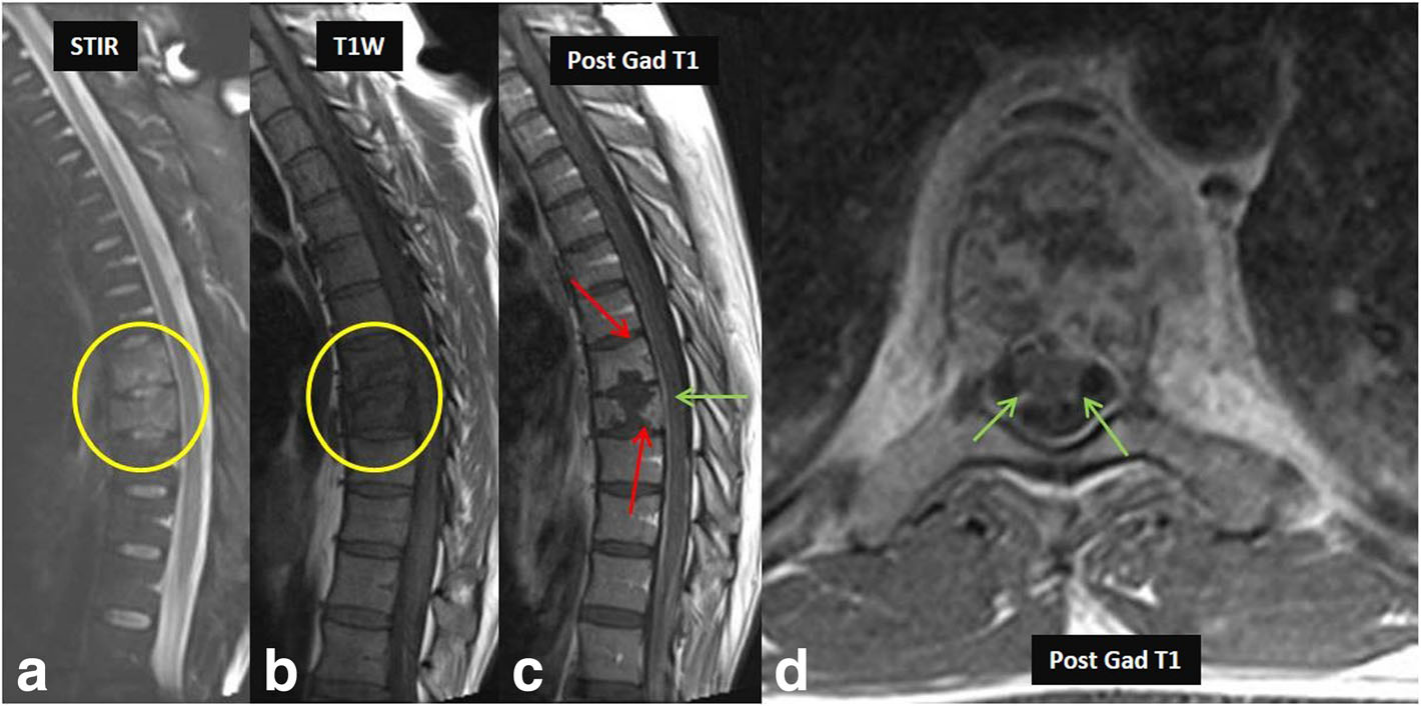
27-year-old-male patient who was an immigrant from South America presented with back pain. Initial MRI was normal. Follow up MRI of the thoracolumbar spine for persistent back pain revealed marrow edema in the lower thoracic spine (*yellow circle* in a and b). Post contrast imaging showed discitis-osteomyelitis complex (*red arrows* in c). Note the enhancement of nerve roots suggesting extension of infectious process to the nerve roots causing arachnoiditis (*green arrows* in c and d)
